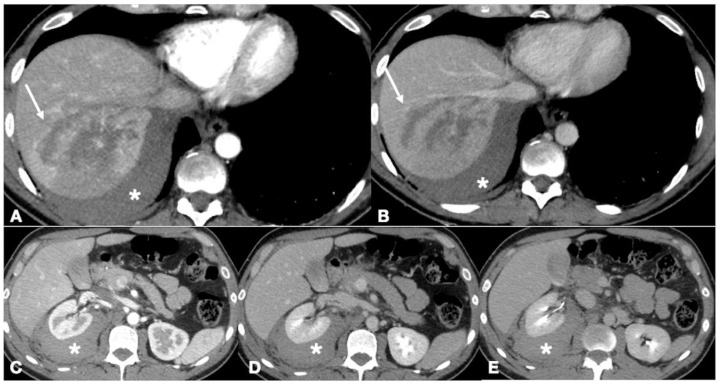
Enhanced-CT of a 37-year old male with multiple injuries due to high energy blunt trauma (car accident). The CT study demonstrates hemoperitoneum (A,B, asterisks), liver lacerations (A, arterial phase, arrow; B, portal venous phase, arrow) and a perirenal hematoma (C–E asterisks). The availability of multiple phases excluded the presence of active bleeding or active urine extravasation. The patient was managed conservatively.
